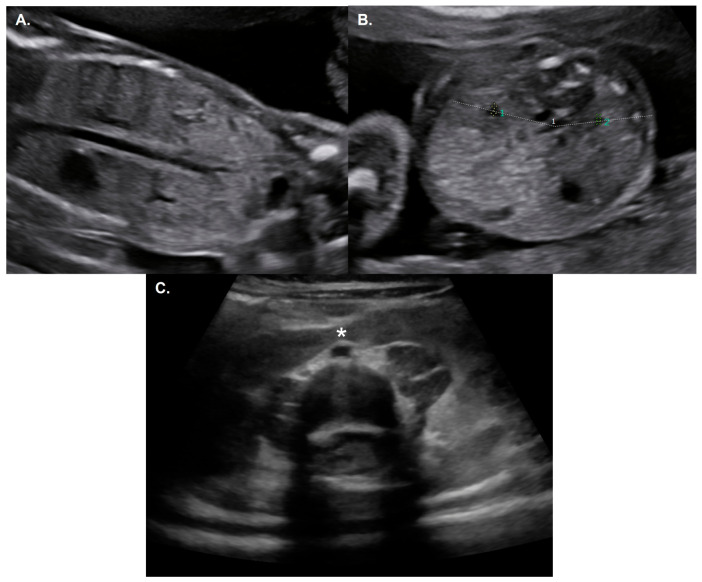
A representative case from Group 1: Postnatal diagnosis of horseshoe kidney (HSK) following unremarkable prenatal ultrasonographic findings. (A) Prenatal gray-scale ultrasonography at 20 weeks of gestation demonstrating no apparent renal anomalies in the coronal view. (B) Measurement of the renal pelvic angle (160°), a finding highly suggestive of normal renal development during the prenatal period. (C) Definitive postnatal diagnosis of HSK confirmed during clinical evaluation in the neonatal intensive care unit. Asterisk (*) indicates the renal isthmus, which was not clearly identified during the prenatal scan. HSK, horseshoe kidney.
